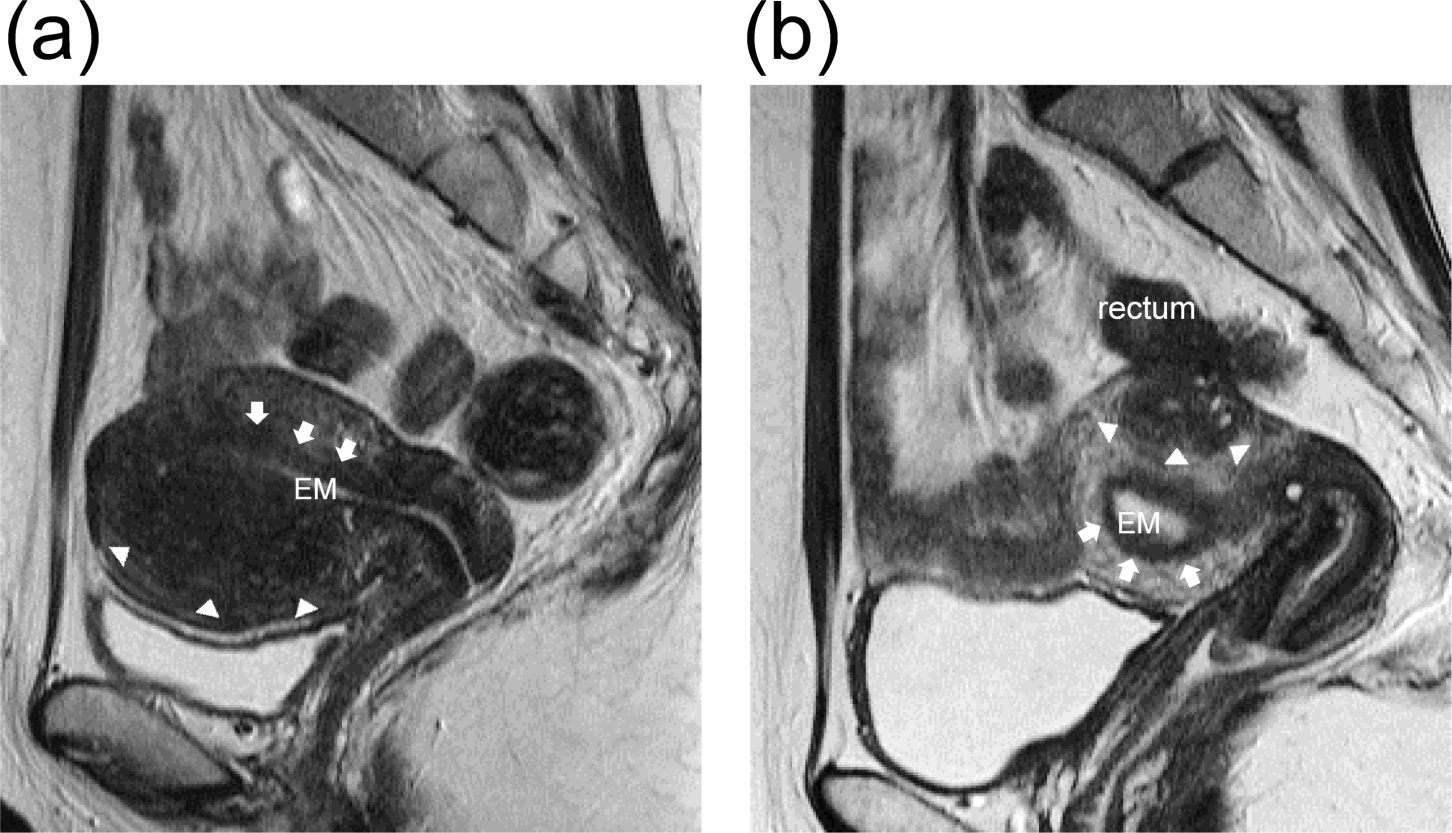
(a) Subtype I adenomyosis. T2-weighted magnetic resonance image (sagittal section) of a uterus with Subtype I adenomyosis. The endometrium (EM) is seen as a T2 high intensity band at the inner most part of the uterus. Adenomyosis resides at the inner myometrium with a direct connection to the junctional zone (JZ). The JZ is a T2 low intensity band surrounding the endometrium (arrows). Adenomyotic foci are seen as a T2 low intensity areas with small high intensity spots in the myometrium. The border between the adenomyotic foci and the outer myometrium are indicated by arrow heads. The outer myometrium is preserved and intact. This image was taken for a 39-year old multiparous woman. (b) Subtype II adenomyosis. T2-weighted magnetic resonance image (sagittal section) of a uterus with Subtype II adenomyosis. Adenomyosis resides in the posterior outer myometrium without affecting the JZ (arrows). The border between the adenomyotic foci and the surrounding myometrium is indicated by arrows. The healthy muscle structures are seen in between the adenomyosis and the JZ. Utero-rectal adhesion caused by pelvic endometriosis is suspected. This image was taken for a 30-year old nulliparous woman.
